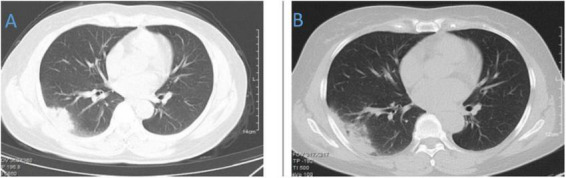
Chest CT of patient 7. (A) Before treatment on 2021.4.19. (B) The follow-up imaging 1 week after treatment showed progression compared to the pre-treatment scans.
